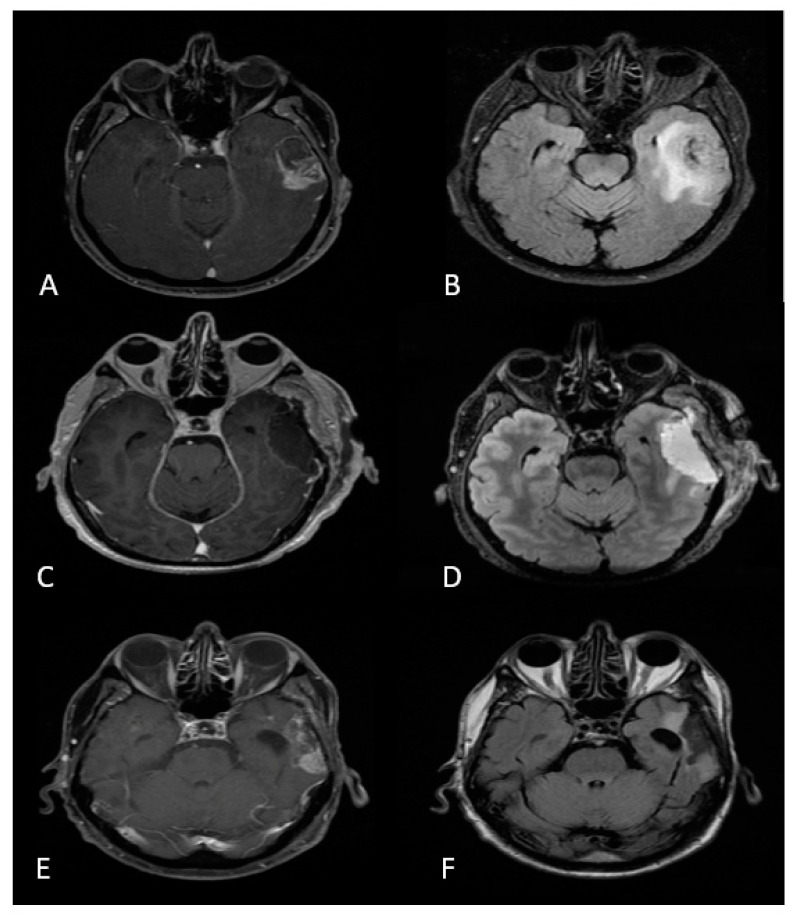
71-year-old man with left temporal glioblastoma. (A,B) Preoperative T1 post-contrast and T2 FLAIR MRI demonstrating large ring enhancing left temporal lesion. (C,D) Postoperative T1 post-contrast and T2 FLAIR MRI highlighting gross total resection of the contrast enhancing lesion, with some minimal residual FLAIR signal. (E,F) 11-month postoperative T1 post-contrast and T2 FLAIR MRI showing a contrast enhancing lesion in the left temporal lobe near the resection cavity consistent with recurrence.
